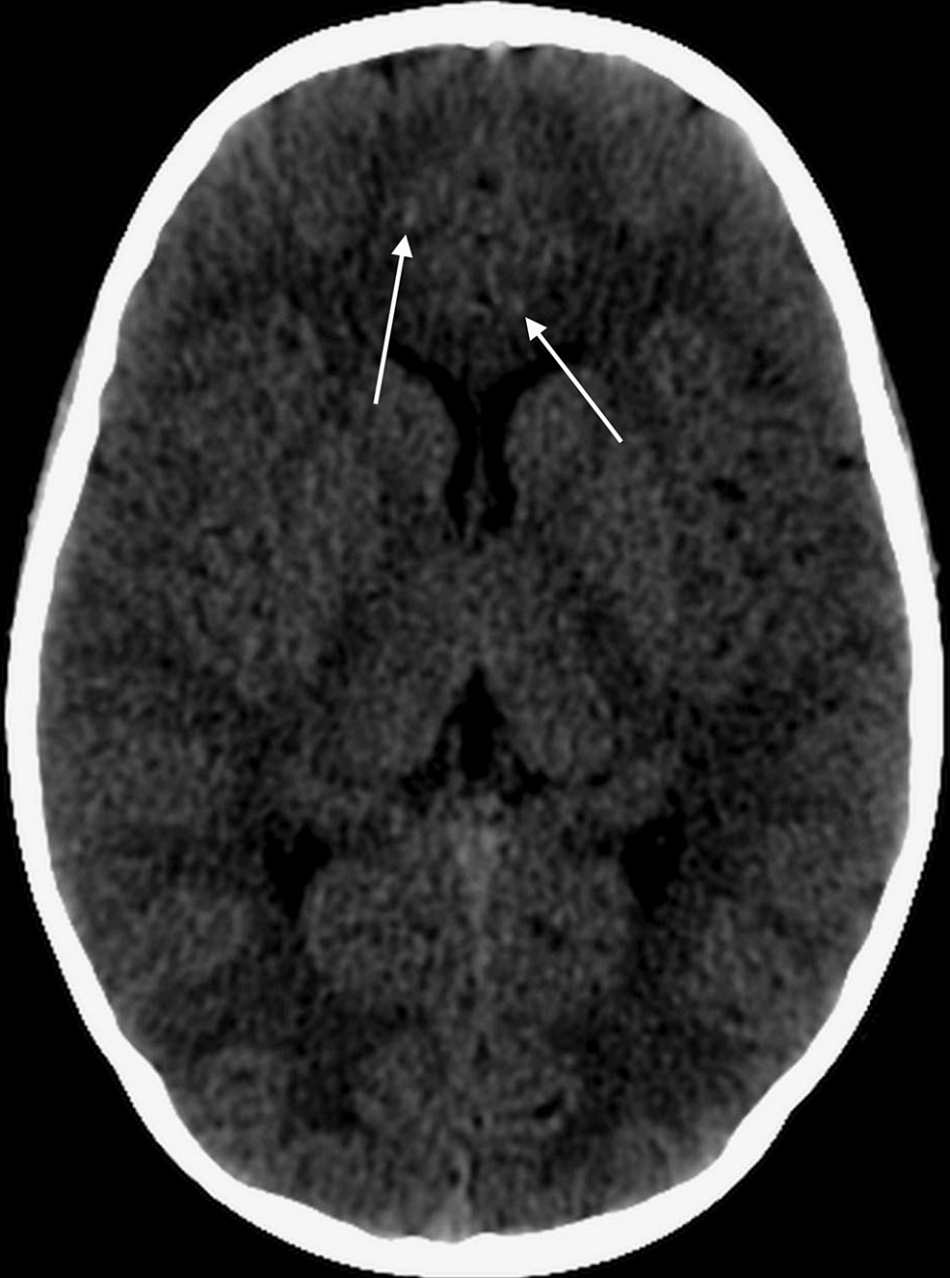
The brain computed tomography (CT) examination demonstrates the presence of discrete bilateral subcortical focal areas exhibiting subtle hyperdensity within the frontal lobes of the brain. These focal regions exhibit discreet calcific deposits, giving rise to localized enhancements in radiodensity within the cerebral parenchyma.
